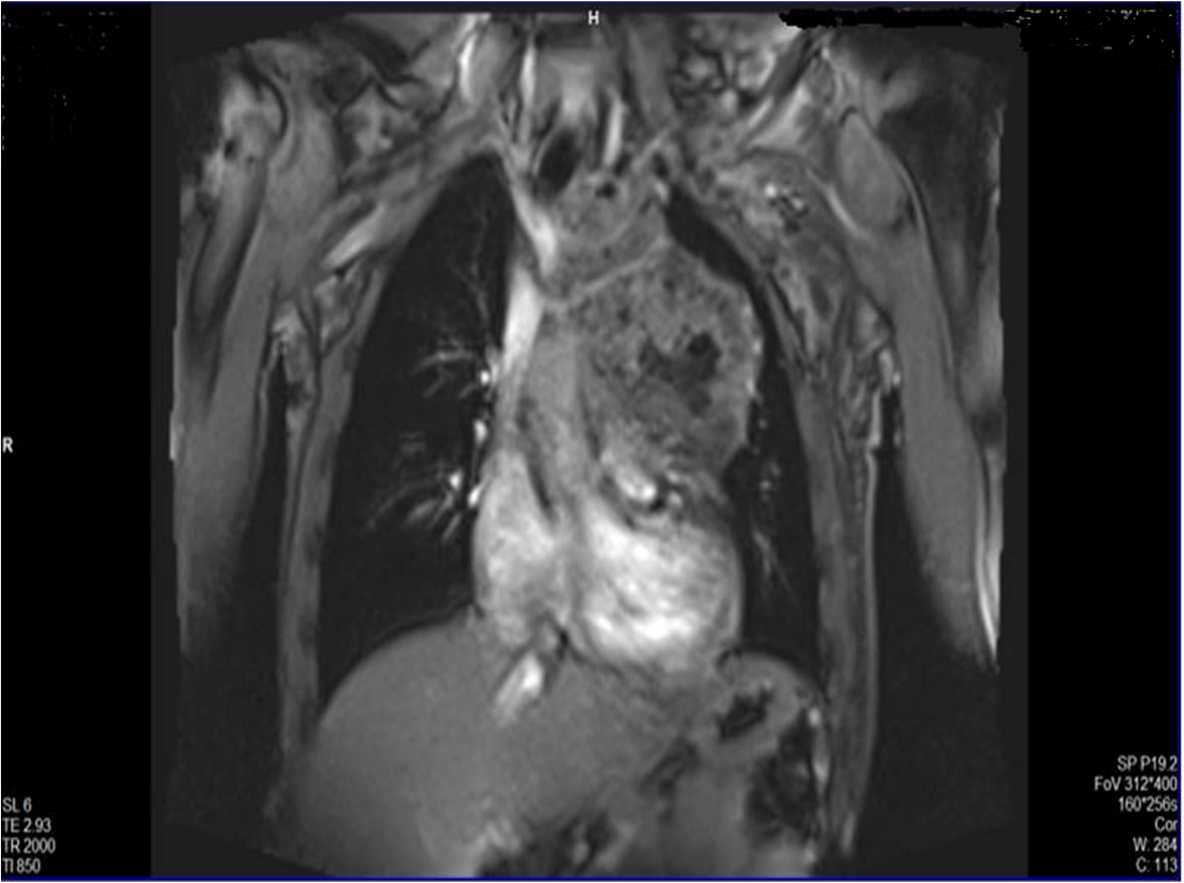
Magnetic resonance imaging of the chest showing the anterior mediastinal mass near the pulmonary artery
